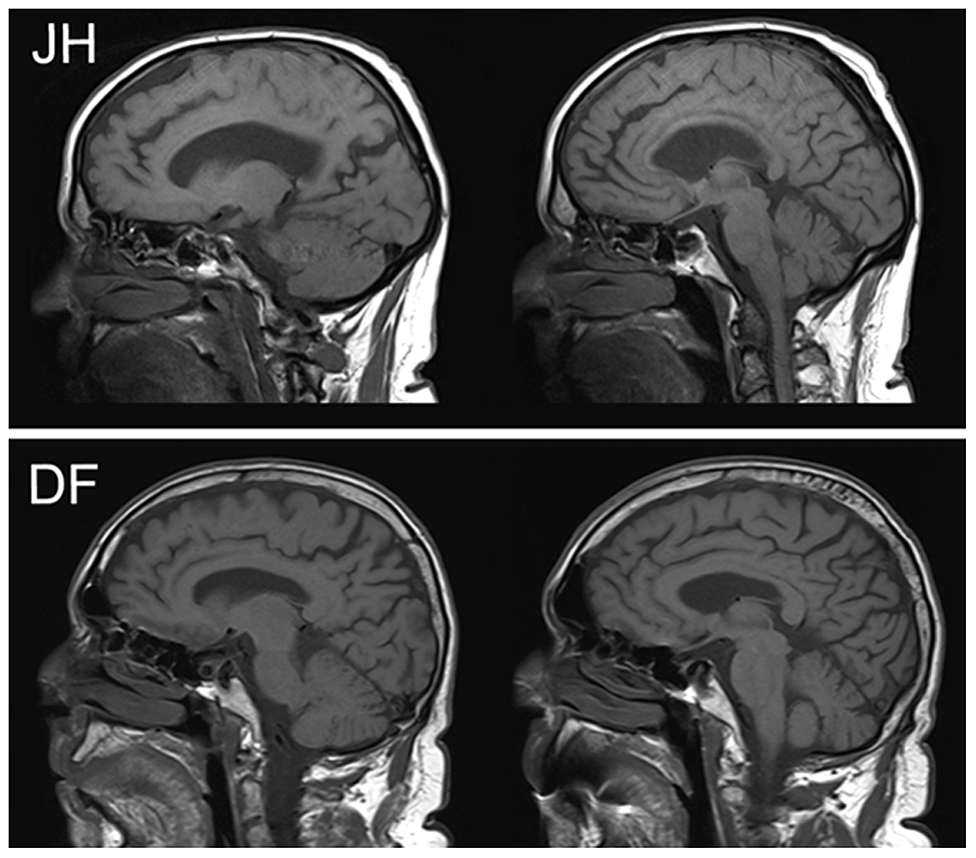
Clinical MRI images in sagittal plane for patients JH (above) and DF (below). There is visible atrophy in the dorsal aspects of mPFC for JH but not DF.
